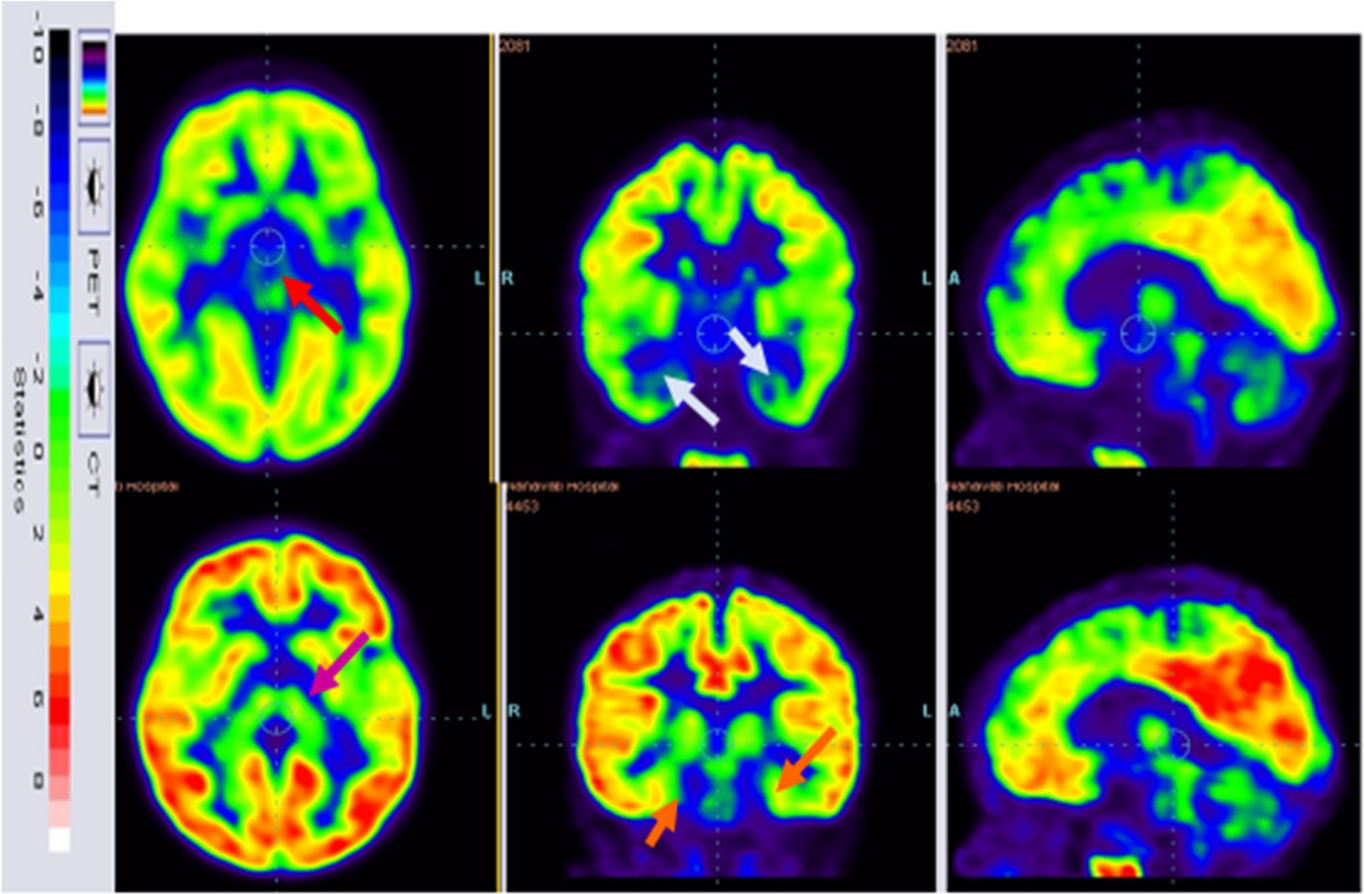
Top row: 18 F-FDG image before cellular therapy showing reduced metabolism in the thalamus (red arrow) and mesial temporal structures (white arrows). Bottom row: improved 18 F-FDG metabolism after cellular therapy metabolism in the thalamus (pink arrow) and mesial temporal structures (orange arrows). CT computed tomography, PET positron emission tomography
